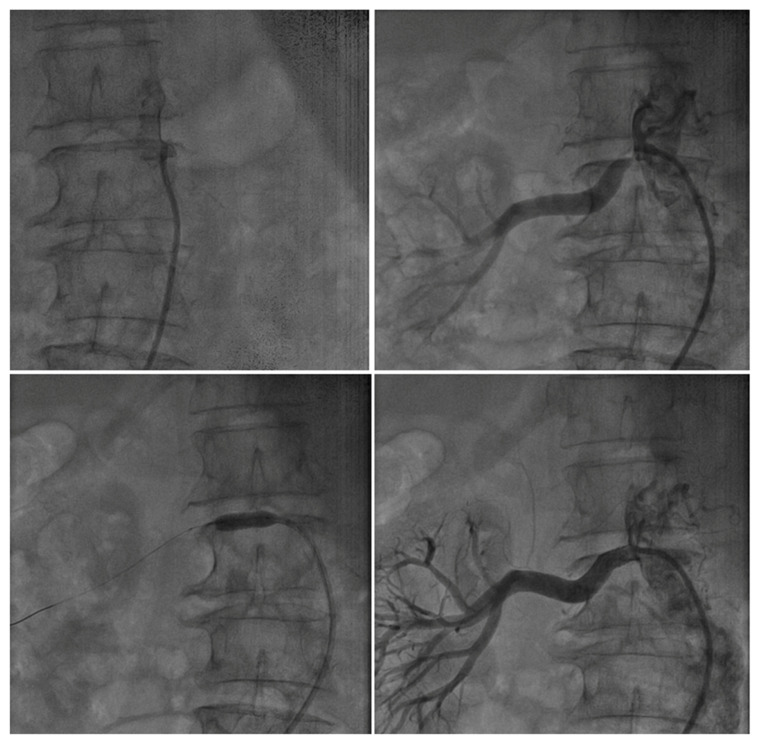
A 73-year-old female patient. Comorbidities included HT, DM, CAD (history of multiple PCI procedures), and CKD. Recent history of emergency department presentation due to HT and HF-related AC edema. Creatinine levels have elevated from 1.3 to 1.6 over the past month. Bilateral selective renal angiography revealed 95–99% stenosis in the right renal ostium and total occlusion of the left renal artery. CT angiography showed the left kidney was atrophic, with no perfusion observed; no blood flow was detected; therefore, no intervention was performed for the total occlusion of the left renal artery. Pre-dilatation of the right renal artery stenosis was performed with a 4.0 × 12 mm balloon, followed by implantation of a 6.0 × 14 mm balloon-expandable renal stent at 14 atm pressure. Patience was achieved.
